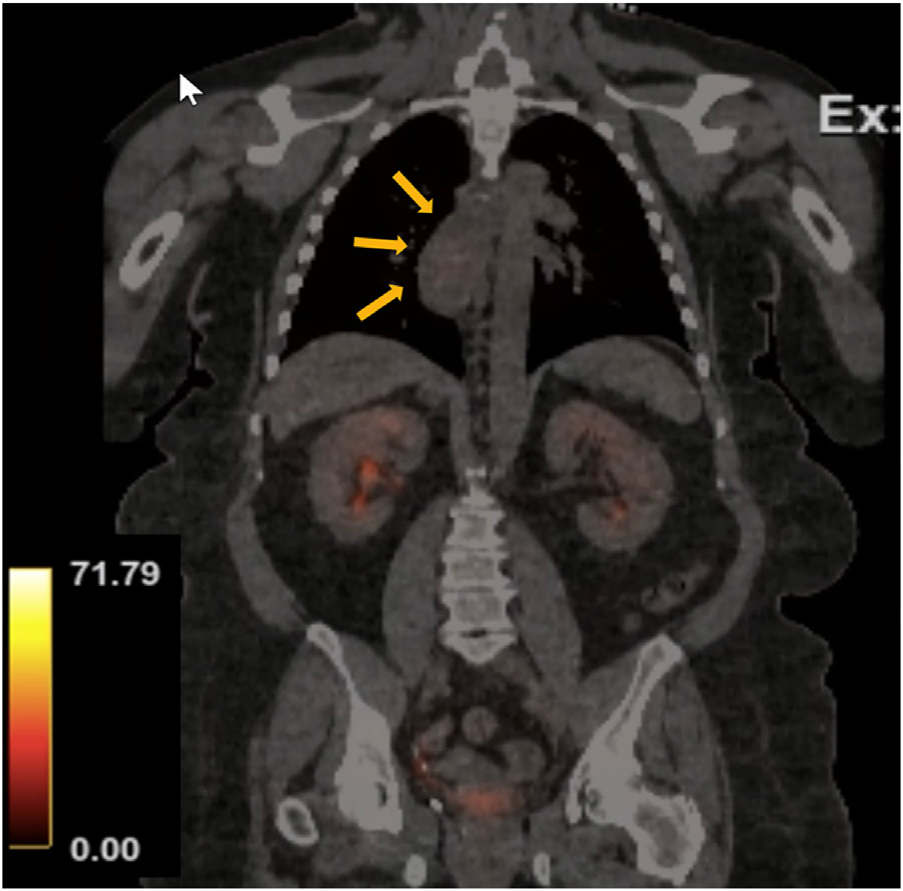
Preoperative positron emission tomography/computed tomography scan with no avidity (arrows highlighting irregularity).
